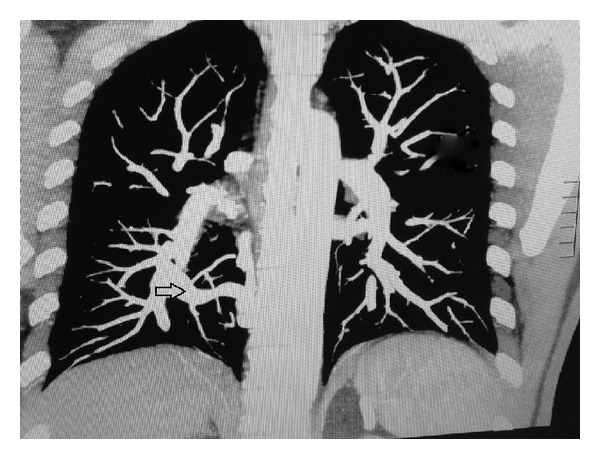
Coronal plane CTA showing fistulous connection between anomalous vein and right inferior pulmonary vein.
